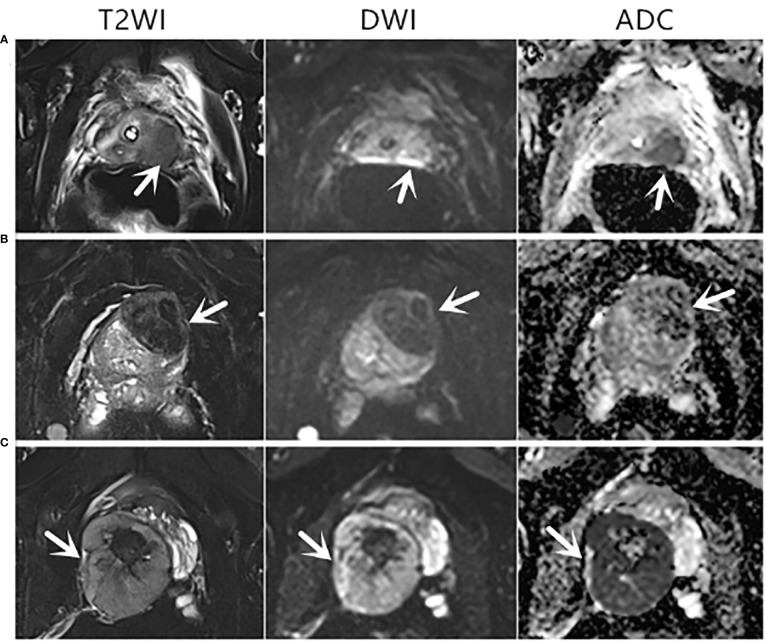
Axial mpMRI images of rare prostate tumor lesions. The mpMRI imaging of three patients who were diagnosed with rare prostate tumors. The PI-RADS score of three patients was 5. (A) An 82-year-old patient with a PSA level of 0.37 ng/ml and urothelial carcinoma revealed by biopsy pathology. (B) A 68-year-old patient with a PSA level of 1.24 ng/ml and a solitary fibrous tumor revealed by biopsy pathology. (C) A 31-year-old patient with a PSA level of 0.73 ng/ml and sarcoma revealed by biopsy pathology. T2WI, T2-weighted image; DWI, diffusion-weighted imaging; ADC, apparent diffusion coefficient.
